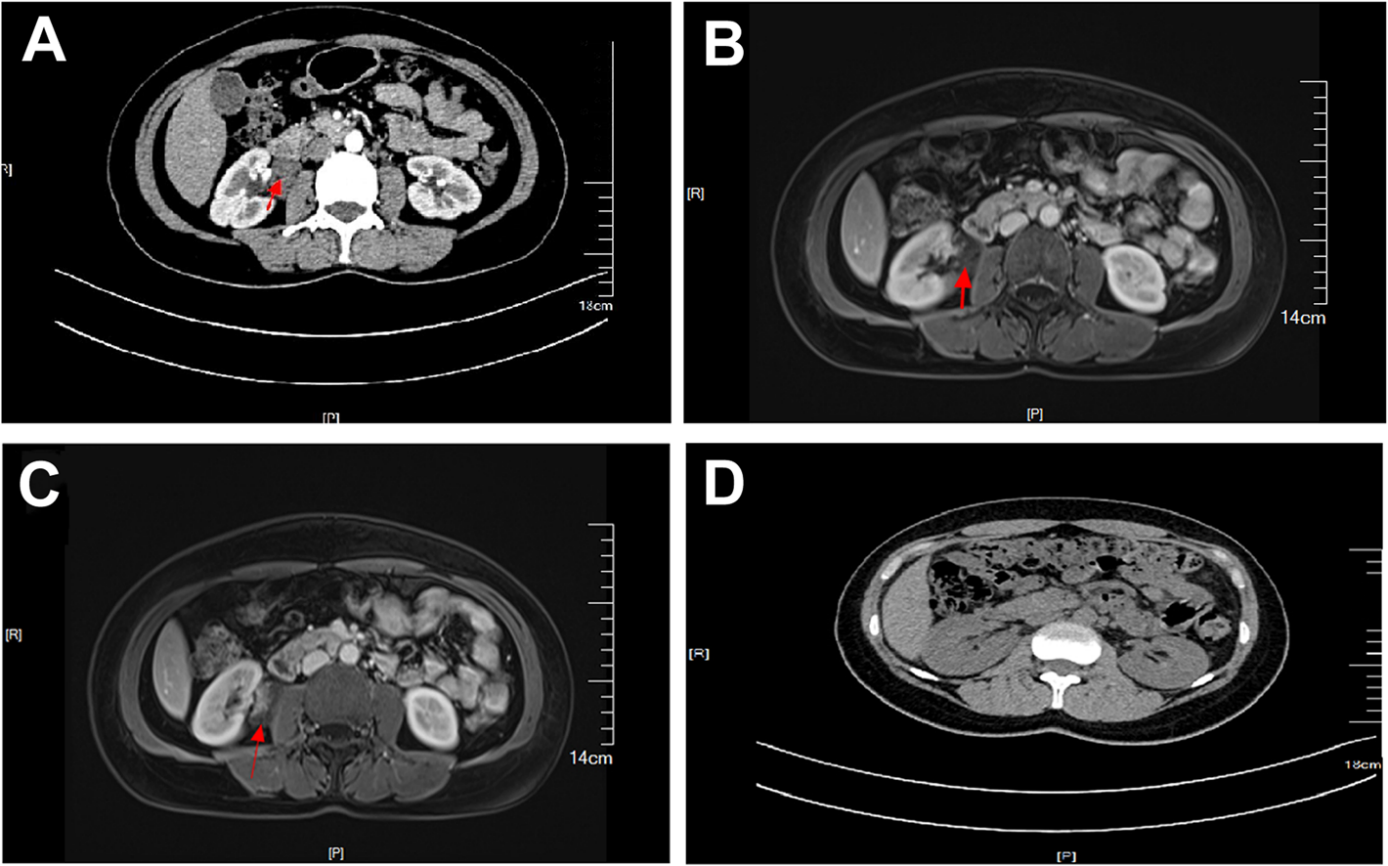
a The CT image of cavernous hemangioma; b The MRI image of cavernous hemangioma; c The enhancement scan image of the cavernous hemangioma; d The CT image of the patient after discharging 3 months
